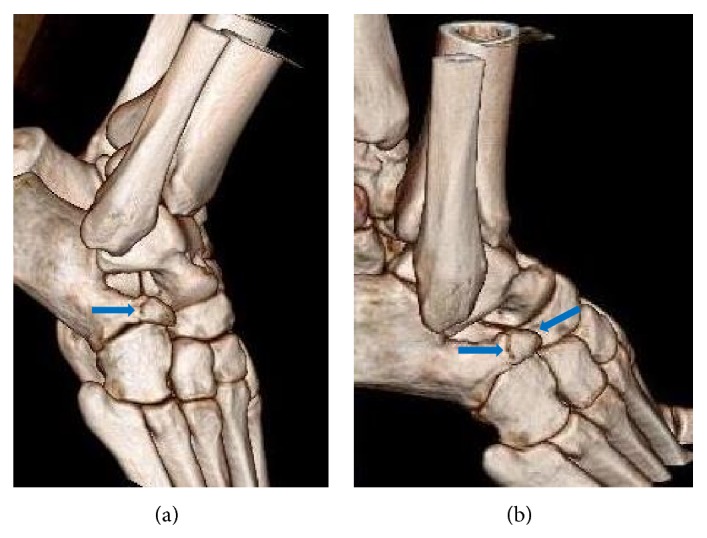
3D volume rendering CT images (a and b) demonstrate os calcaneus secundarius (blue arrows).
